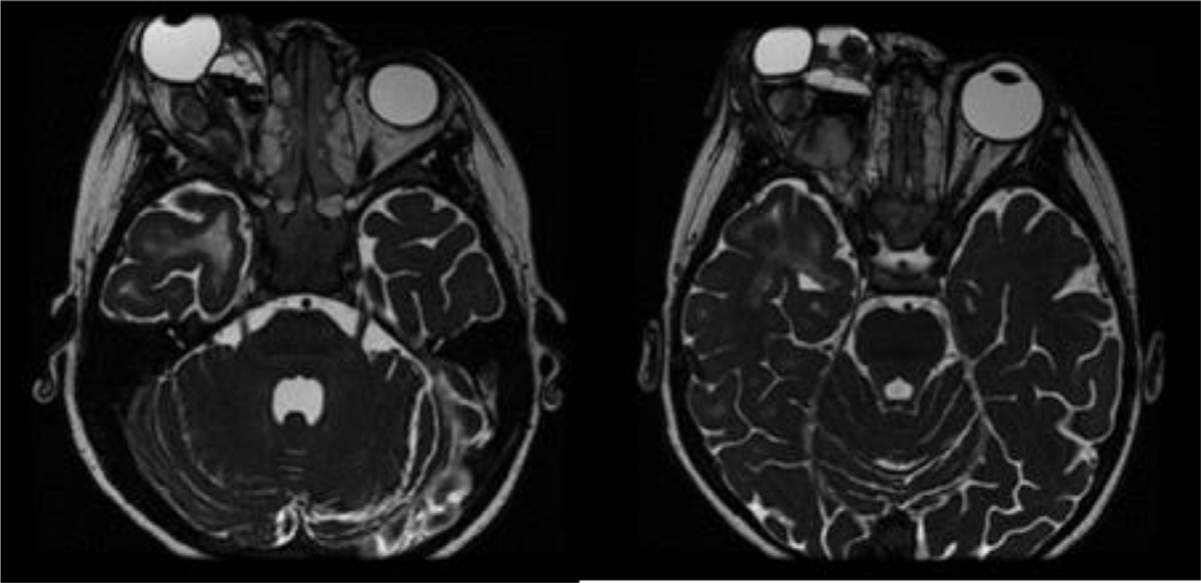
MRI presclerotherapy showed inhomogeneous lesion in right retrobulbar which infiltrated right intraconal and extraconal fat, right nerve optic, and right superior-medial-anterior recti. The distance between the anterior margin of the right ocular bulb and the right interzygomatic line was 2.36 cm.
